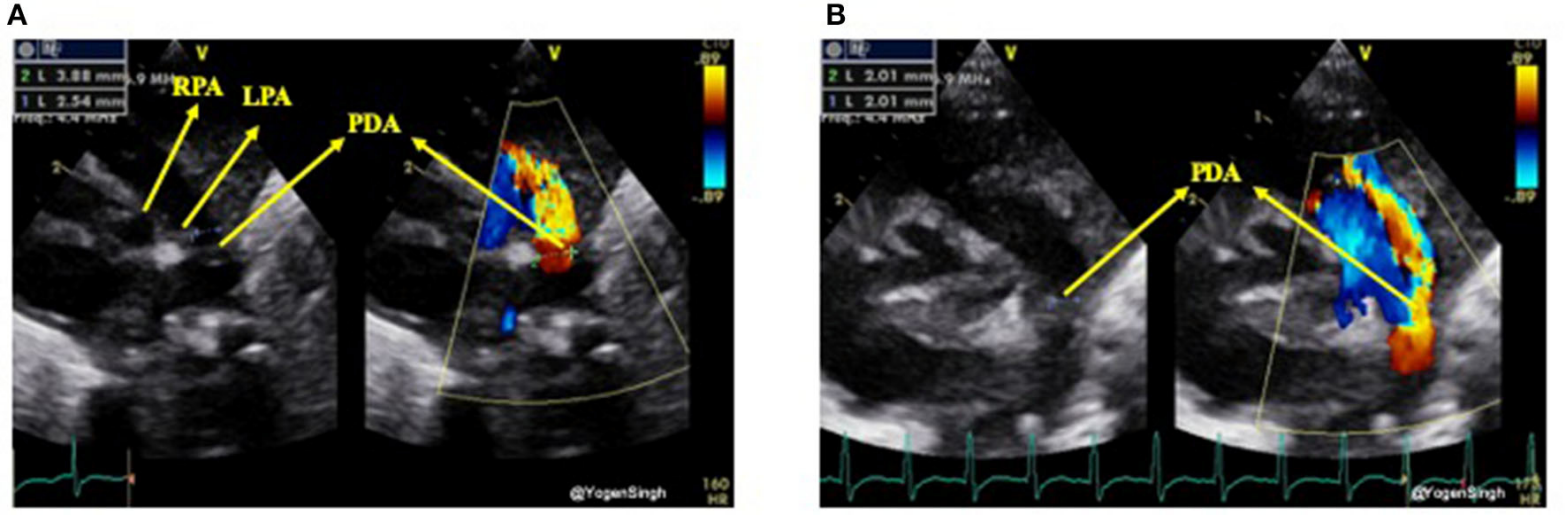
Measurement of ductal size on 2D and color Doppler on high left parasternal “ductal view.” (A) Showing significant discrepency between ductal diameter measurement on 2D and color Doppler—over-estimation of ductal size on color Doppler because of gain setting; (B) showing no significant discrepency between ductal diameter measurement on 2D and color Dopple after optimisation of gain setting. LPA, left pulmonary artery; RPA, right pulmonary artery; PDA, patent ductus arteriosus.
